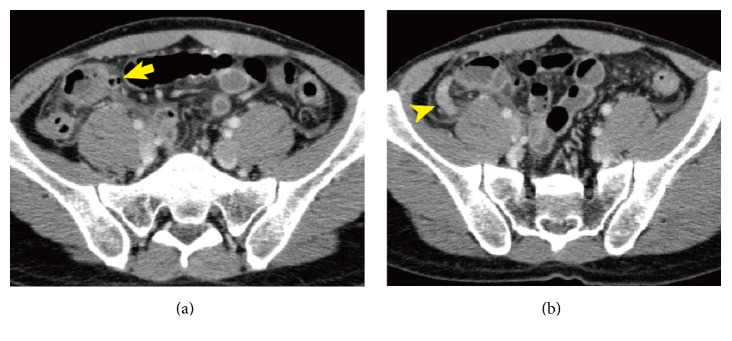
Computed tomography revealed diverticula in the terminal ileum (arrow) and swollen appendix with 10 mm in the diameter (arrow head).
